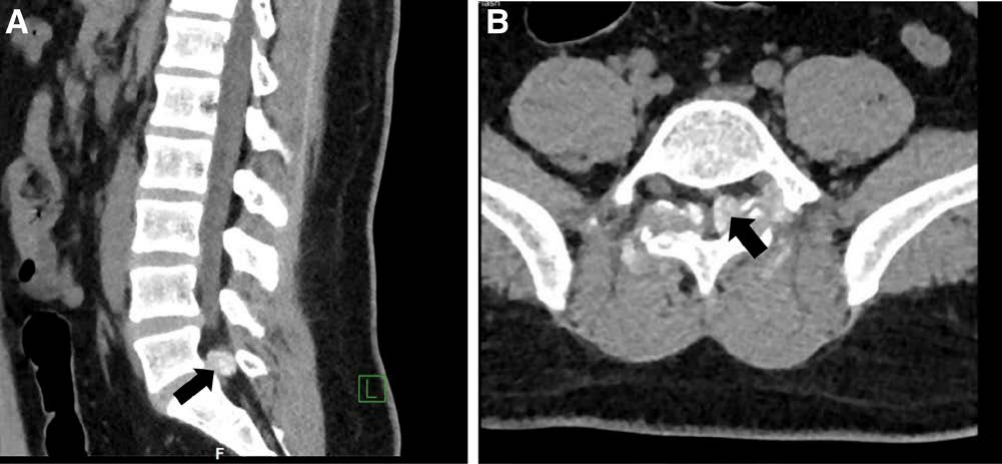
Computed tomography images of the lumbar spine. Note: images revealing partial lesions, round mass within the spinal canal at L5/S1 (indicated by the arrow in the sagittal tomogram [A]); insect erosion and destruction of the bilateral patellar bone in the adnexa of L5, as well as the bilateral involvement of the lower articular processes at L4 and the bilateral articular processes at S1, Corresponding segmental spinal stenosis (marked by an arrow in [B]).
